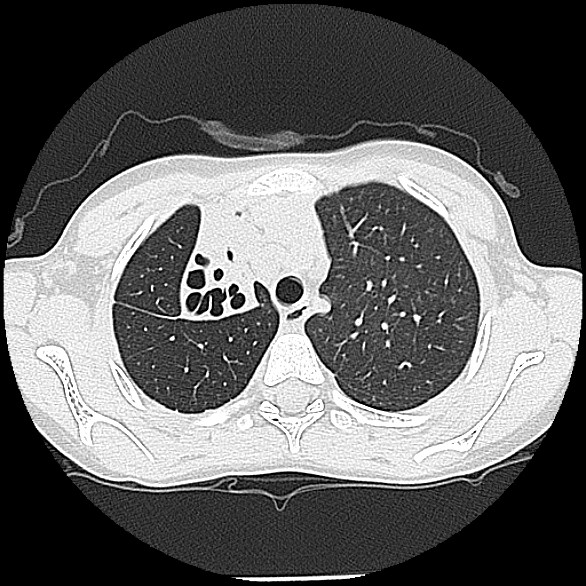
Representative high resolution CT chest slices demonstrating collapse and severe bronchiectasis of the right upper lobe.
